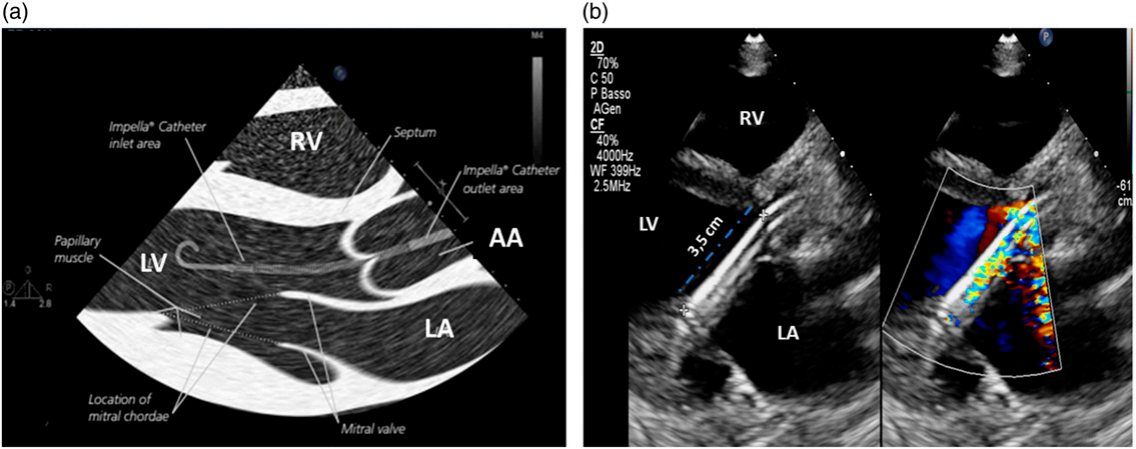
(a) Work-art representation of the ultrasound anatomy a transthoracic parasternal long-axis view of the left ventricle (courtesy of Abiomed, Danvers, MA, USA); (b) Transthoracic parasternal long-axis view of the left ventricle: evaluation of the Impella position (3.5 cm distance from the tear drop to the aortic annulus). RV: right ventricle, LV: left ventricle; LA: left atrium; AA: ascending Aorta.
